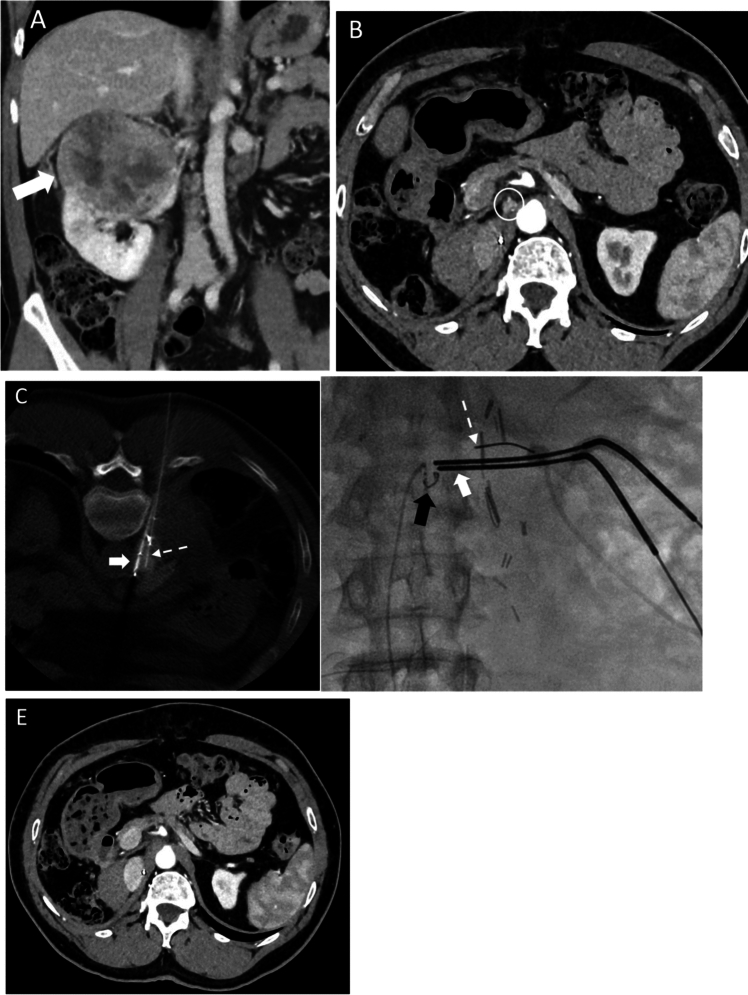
A 67-year-old woman with asynchronous right paraaortic nodal RCC metastasis treated with cryoablation, allowing delay of initiation of immunotherapy. a Coronal reformatted contrast-enhanced CT abdomen showed primary right upper pole RCC (white arrow), which was resected by radial nephrectomy. Pathology showed clear cell RCC. b Axial contrast-enhanced CT abdomen performed 1 year after right nephrectomy showed right paraaortic nodal metastasis (white circle), c and d Under (c) CT and (d) fluoroscopic guidance, cryoablation of the right paraaortic nodal metastasis performed using two 17G cryoprobes (white arrow). A 22G spinal needle (dashed white arrow) was used for hydrodissection. Coil embolisation of a parasitic right diaphragmatic artery supplying the node also performed to reduce cold sink effect. e axial contrast-enhanced CT at 6 months post-cryoablation confirmed necrosis of nodal metastasis
